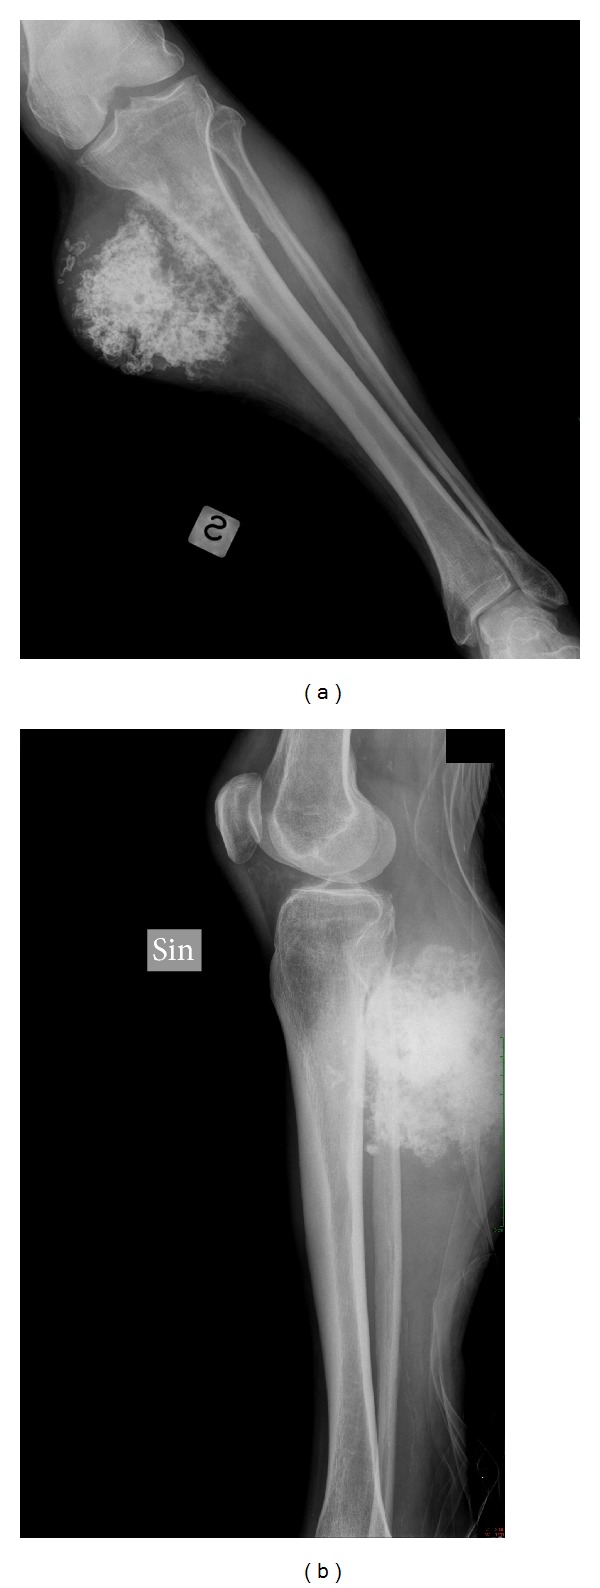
(a) AP and (b) lateral radiographs of tibia and fibula demonstrating a peripheral dedifferentiated chondrosarcoma with mineralization and a soft-tissue mass.
